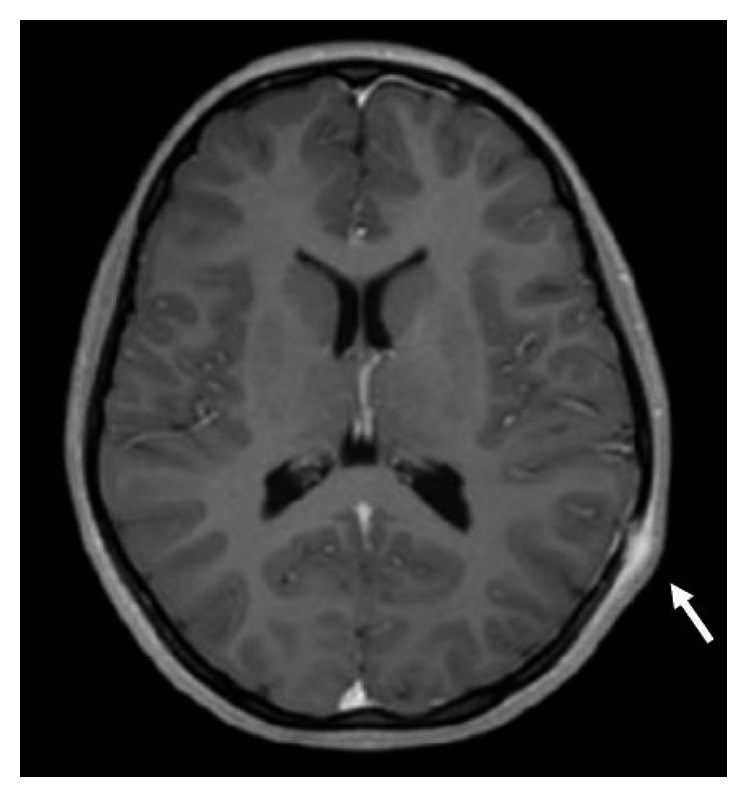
Cranial magnetic resonance imaging with contrast: in the left temporoparietal area in correspondence with clinical painful swelling is present an erosion of the skull of about 10 mm. The external periosteum is thickened with marked contrast enhancement in a 4 cm area.
